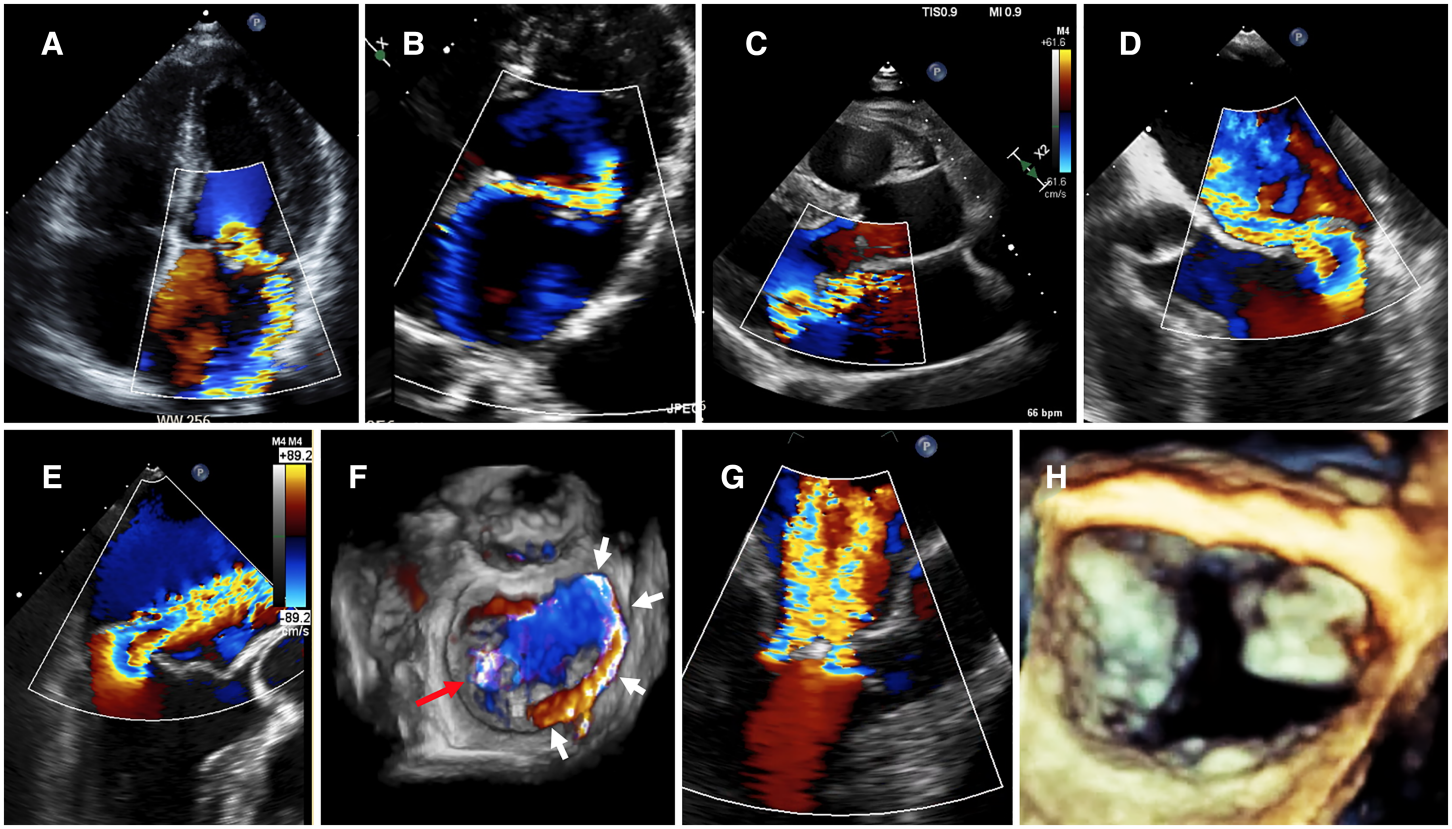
Doppler color approach of primary MR evaluation. (A) Anterior MV prolapse with eccentric MR jet impinging on the posterior LA wall because of Coandă effect. The direction of the MR jet helps in understanding the prolapse's mechanism. (B) Posterior MV prolapse with eccentric jet impinging on the interatrial septum down to the pulmonary veins. Despite a small color flow jet area, a significant MR should be suspected because of the presence of a Coandă effect. (C) Posterior MV prolapse with large spontaneous proximal flow convergence (i.e without modifying Doppler color settings). A significant MR should be suspected. (D,E) Examples of posterior MV flail by TEE where the 3 components of the MR are clearly visualized (proximal flow convergence, vena contracta and distal MR jet). Hence, it is possible to measure 2D-vena contracta width. However, it should be kept in mind that the measurement of 2D-VCW could be less reliable when performed orthogonal to the direction of the ultrasound beam because of lower lateral than axial resolution. (F) Surgical “en face” view with Doppler color mode. A full MV 3D color-coded data set can objectively document MR regurgitant(s) jet(s). However, this method could suffer from markedly decreased framerate, therefore multi-beat acquisition is usually required to obtain an acceptable quality imaging. Herein, the origin of the MR is visualized at the MV anterior commissure (red arrow), and the distal MR jet is impinging on the inter-atrial septal wall because of the Coandă effect. (G,H) Double MR jet with a pattern of “Crossed swords sign”. 3D-TEE imaging revealed a large anterior cleft indentation. 3D, three-dimensional; LA, left atrium; MR, mitral regurgitation; MV, mitral valve; TEE, transesophageal echocardiography; TTE, transthoracic echocardiography; VCW, vena contracta width.
